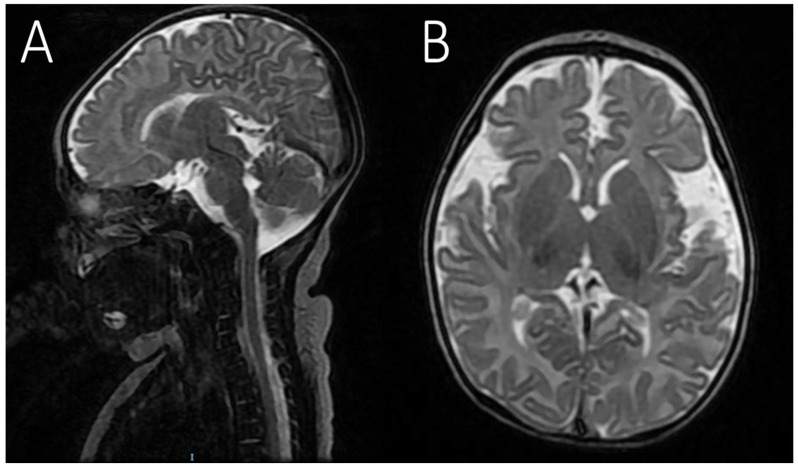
Brain MRI of our patient. (A) Sagittal T2 sequence; (B) Axial T2 sequence showing incomplete myelination, normal for age (10 days). No other abnormalities involving the cerebral, cerebellar, and brainstem parenchyma.
